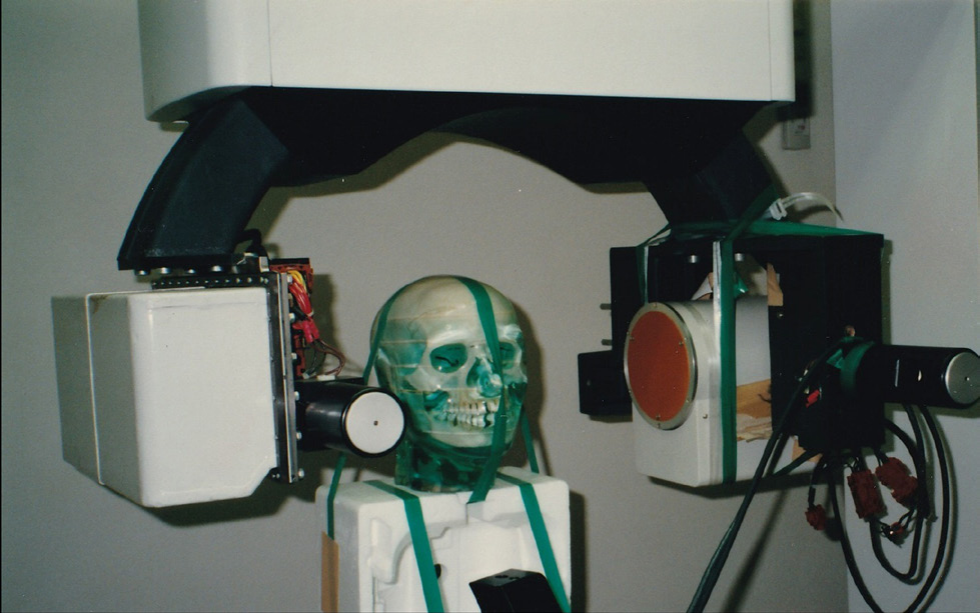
Prototype of local cone beam CT. An image intensifier with a diameter of 10 cm was mounted on the *Scanora^®^*.
